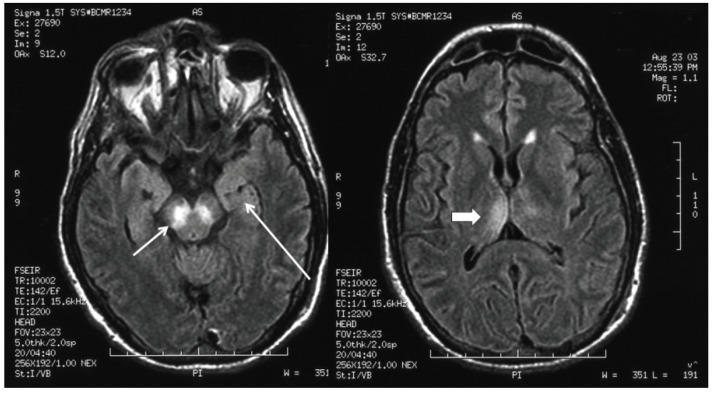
Fluid-attenuated inversion recovery magnetic resonance imaging sequence of the brain in a patient with West Nile virus encephalitis with associated parkinsonism and tremor, displaying signal abnormality in the substantia nigra (short arrow), the mesial temporal lobe (long arrow) and right posterior thalamus (thick arrow).
